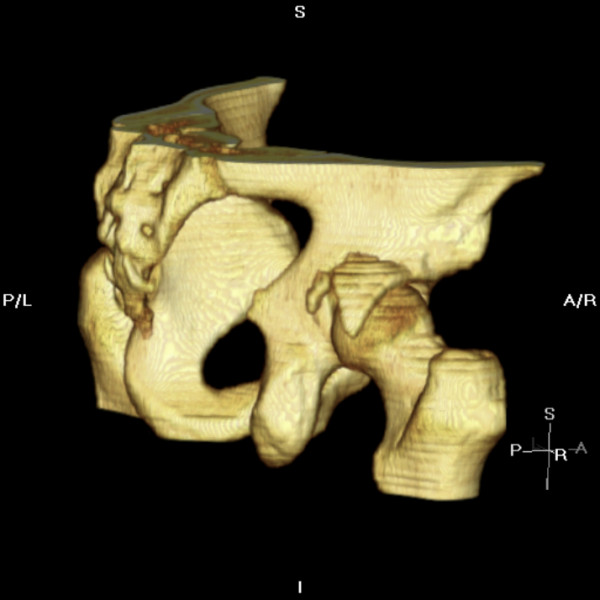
CT scan with 3 D reconstruction.
